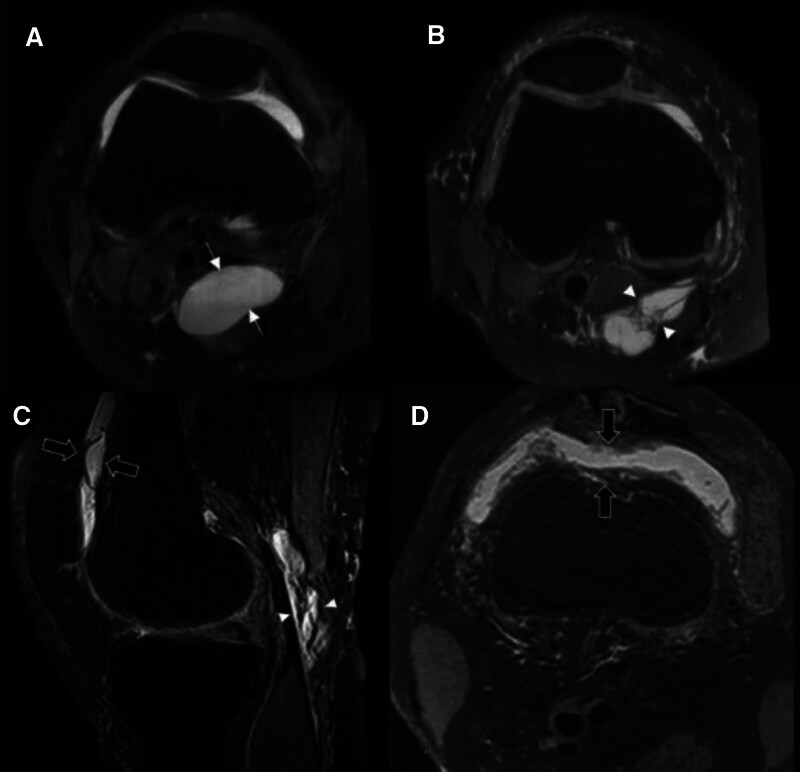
Axial and sagittal view of fat-suppressed proton-density MRI of the BC. (A) Simple BC (arrows) is characterized as homogenous signal intensity lesion with smooth well-defined borders and thin synovial wall. (B, C) Complicated BC (arrowheads) shows internal nonhomogeneity, septation, synovial thickening, and intracystic nodules or debris. (C, D) Synovial proliferation in the suprapatellar recess (black arrows) is observed in the knee with complicated BC. BC = Baker’s cyst, MRI = magnetic resonance imaging.
